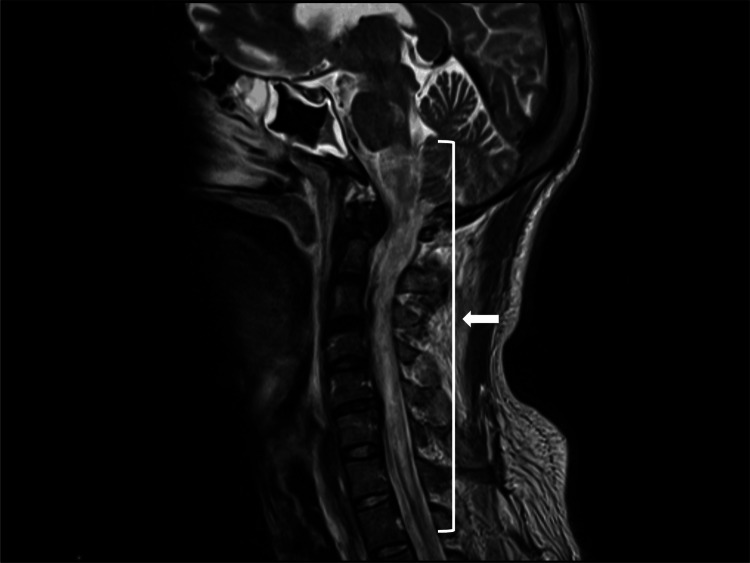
Initial T2-Weighted MRI Findings Sagittal T2-weighted MRI obtained at the first admission in 2011 showed a high-intensity lesion with swelling extending from the medulla oblongata to the Th1 level, indicated by the bracket and white arrow.
